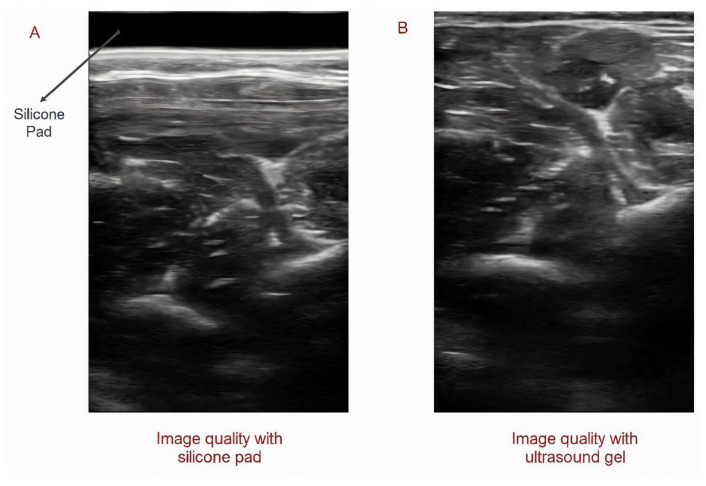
Utilizing silicone pad instead of ultrasound gel: Image quality using silicone pad (A) and ultrasound gel (B).
